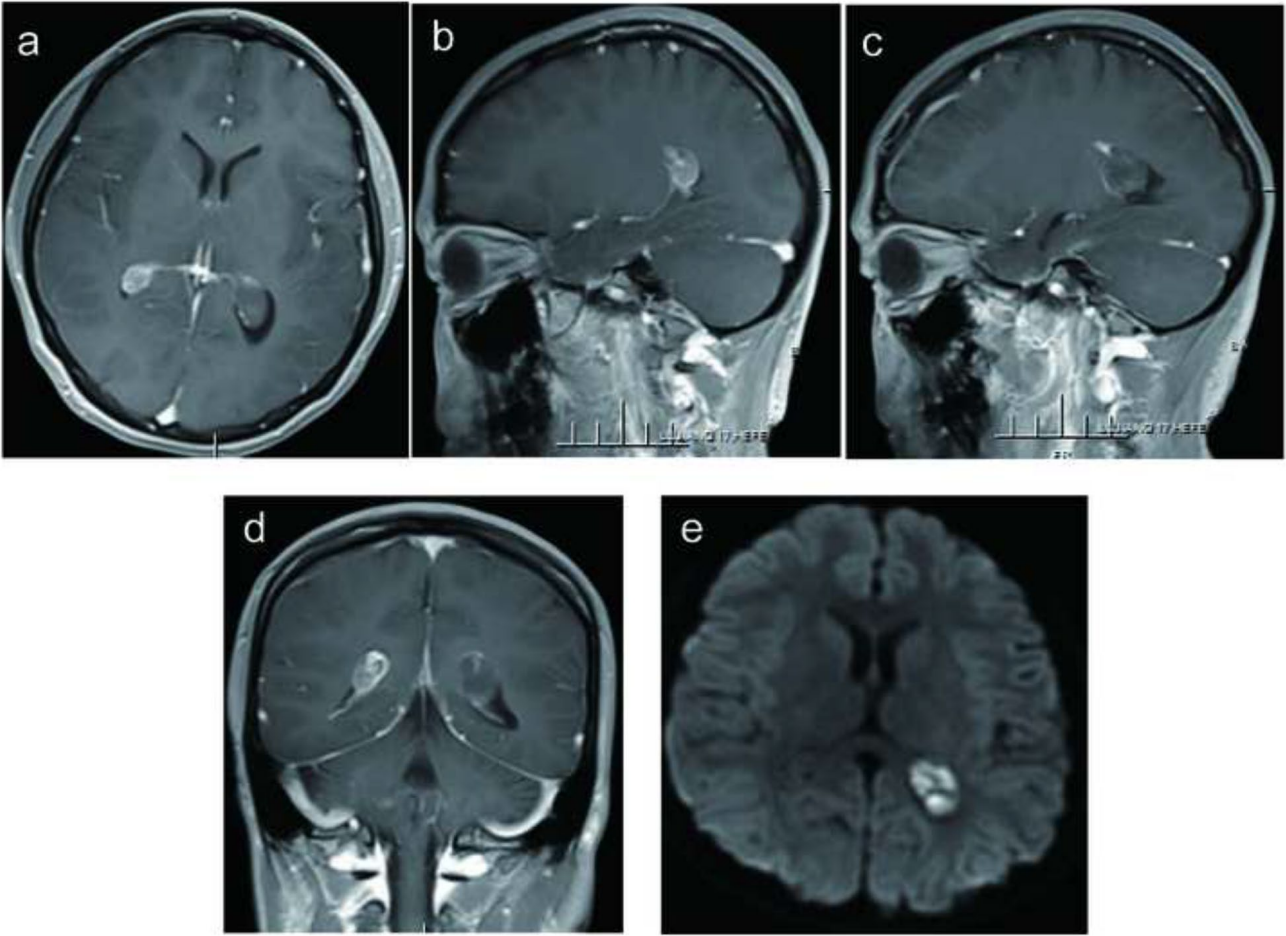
MRI demonstrating multiple CAPNONs. In T1-weighted post-contrast MRI images, there is an absence of contrast enhancement observed in the bilateral lateral ventricles. Please refer to the following horizontal (a), anomalous (b, c), and coronal (d) images; diffusion-weighted imaging (DWI) sequence showed low signal shadow in bilateral lateral ventricle (e)
